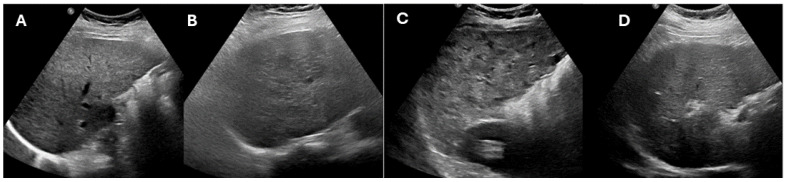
Ultrasound of the liver in four patients with chronic liver disease with the Ultrasound LI-RADS visualization score examples. Image (A) demonstrates coarse hepatic echotexture. The entire hepatic parenchyma is optimally visualized, which corresponds to a US visualization score of A. In image (B), the hepatic parenchyma appears heterogeneous, and evaluation for small hepatic observations is suboptimal. This corresponds to the US visualization score B. In image (C), the hepatic parenchyma is markedly coarsened, severely limiting evaluation for focal hepatic lesions. This corresponds to the US visualization score C. In Image (D), the hepatic parenchyma close to the diaphragm (along the inferior aspect of the image) is suboptimal visualized, which severely limits the evaluation. This corresponds to a US visualization score of C.
